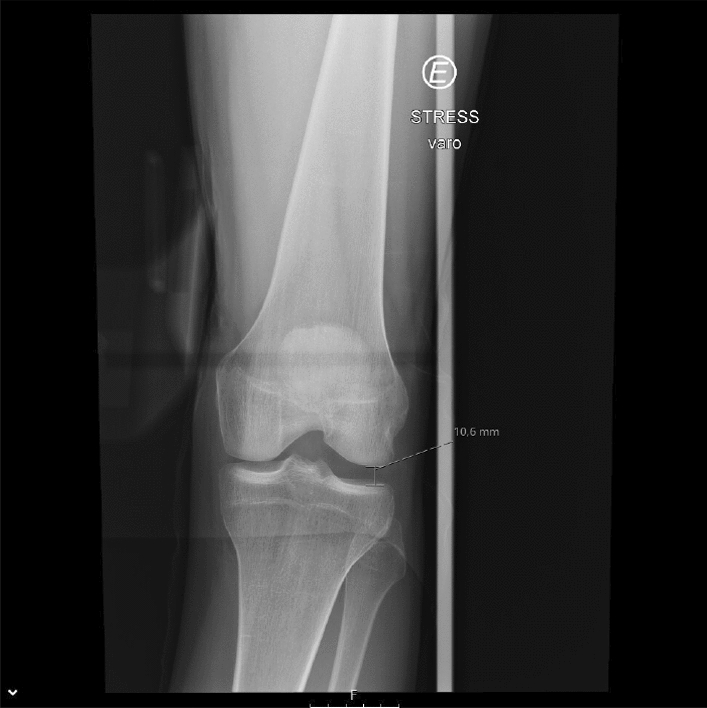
X-ray image with artefact left by the table of examination during the performance of the varus stress test on the left knee (“E” is an indicator for “Left”)
